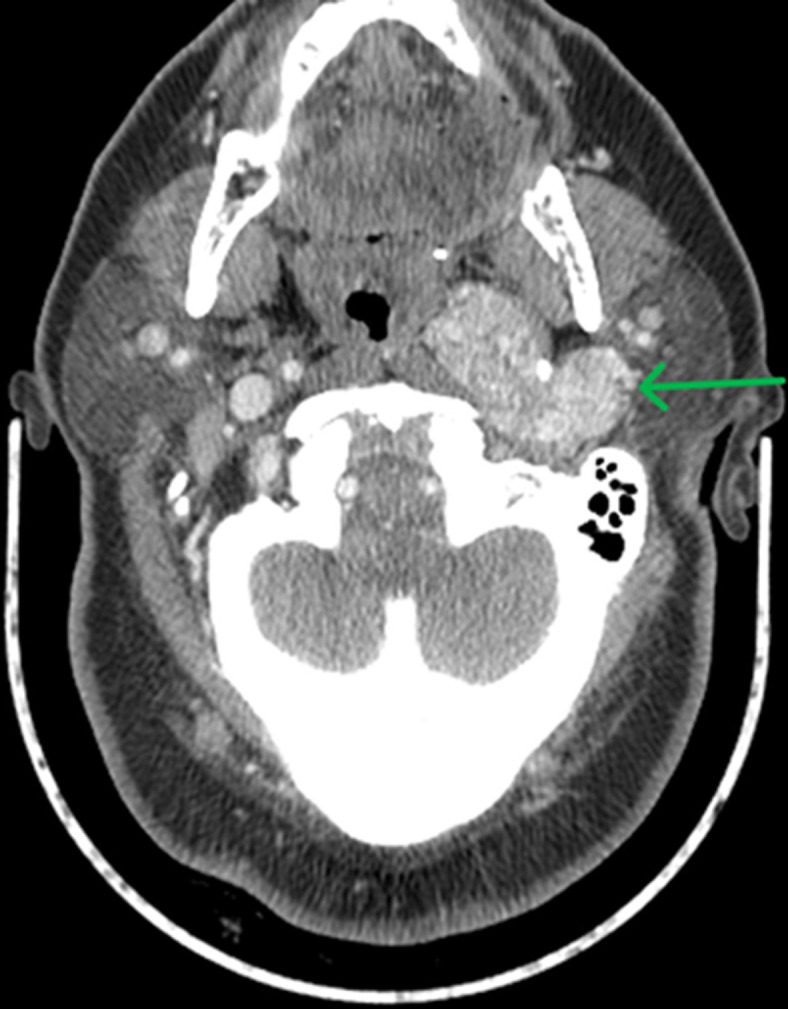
axial CT image of the skull base demonstrating a 6.2 cm hypervascular mass (green arrow) located at the left carotid bifurcation, extending superiorly into and above the left jugular foramen
